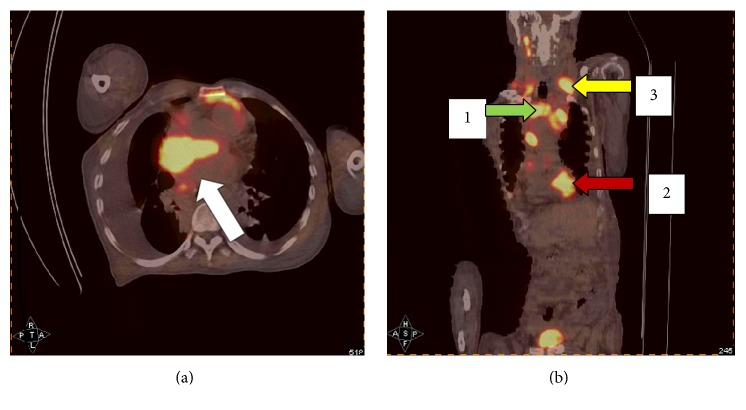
Systemic (18)F-FDG PET/CT scan revealed that the (18)F-FDG uptake in the mass located in the right atria pathologically increased ((a) arrow). Quite a few lymph nodes with increased uptake of (18)F-FDG were revealed at the regions of neck (yellow arrow), mediastinum (green arrow), and right atrium (red arrow) (b).
